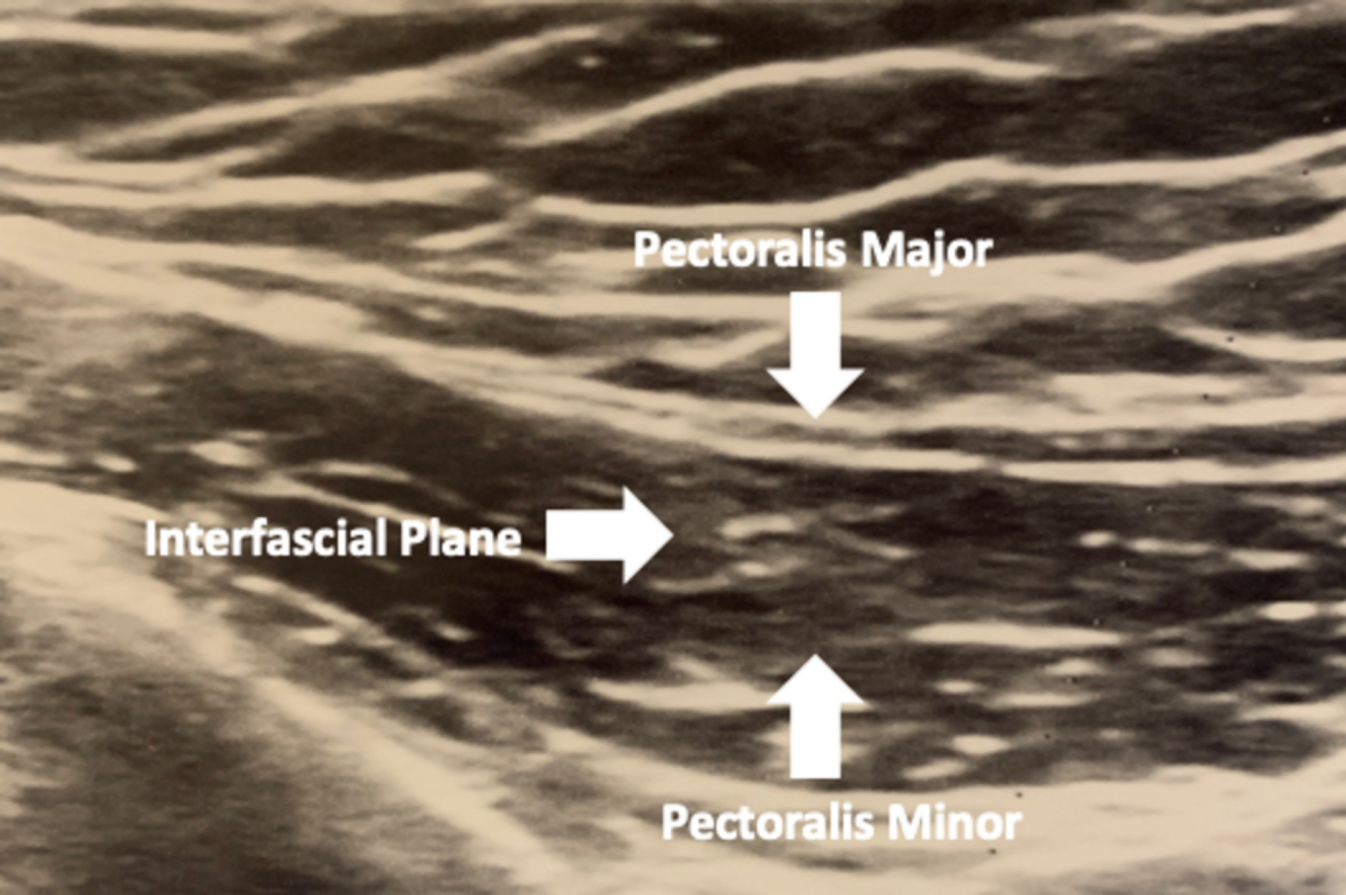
Antero-posterior ultrasound view of the pectoralis major and pectoralis minor muscles.
